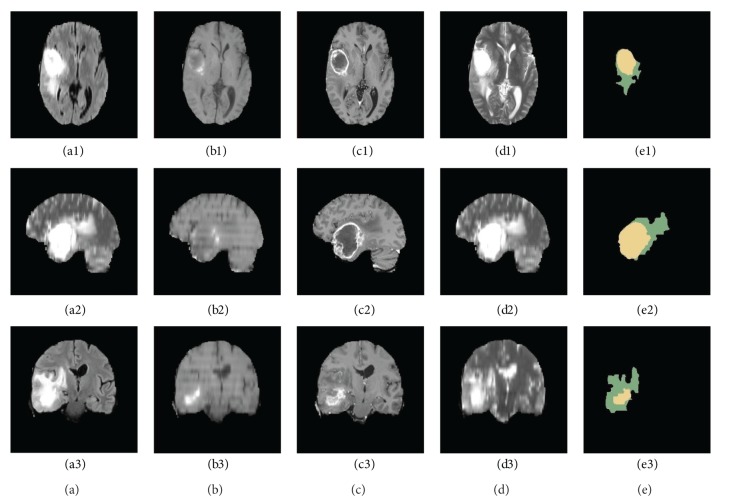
Three-dimensional multimodal slices of clinical high-grade glioma MR images.
